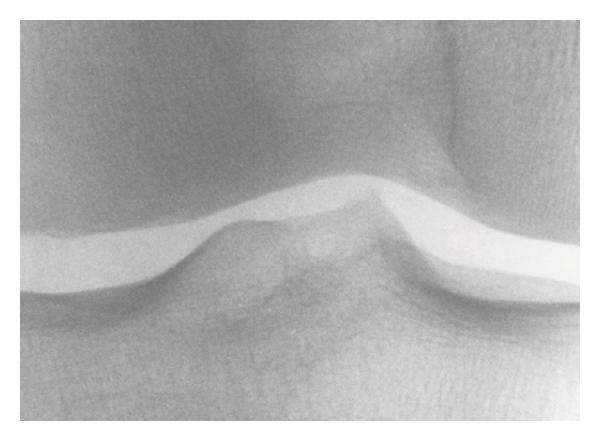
A sample image of a center of a knee X-ray used in this study
